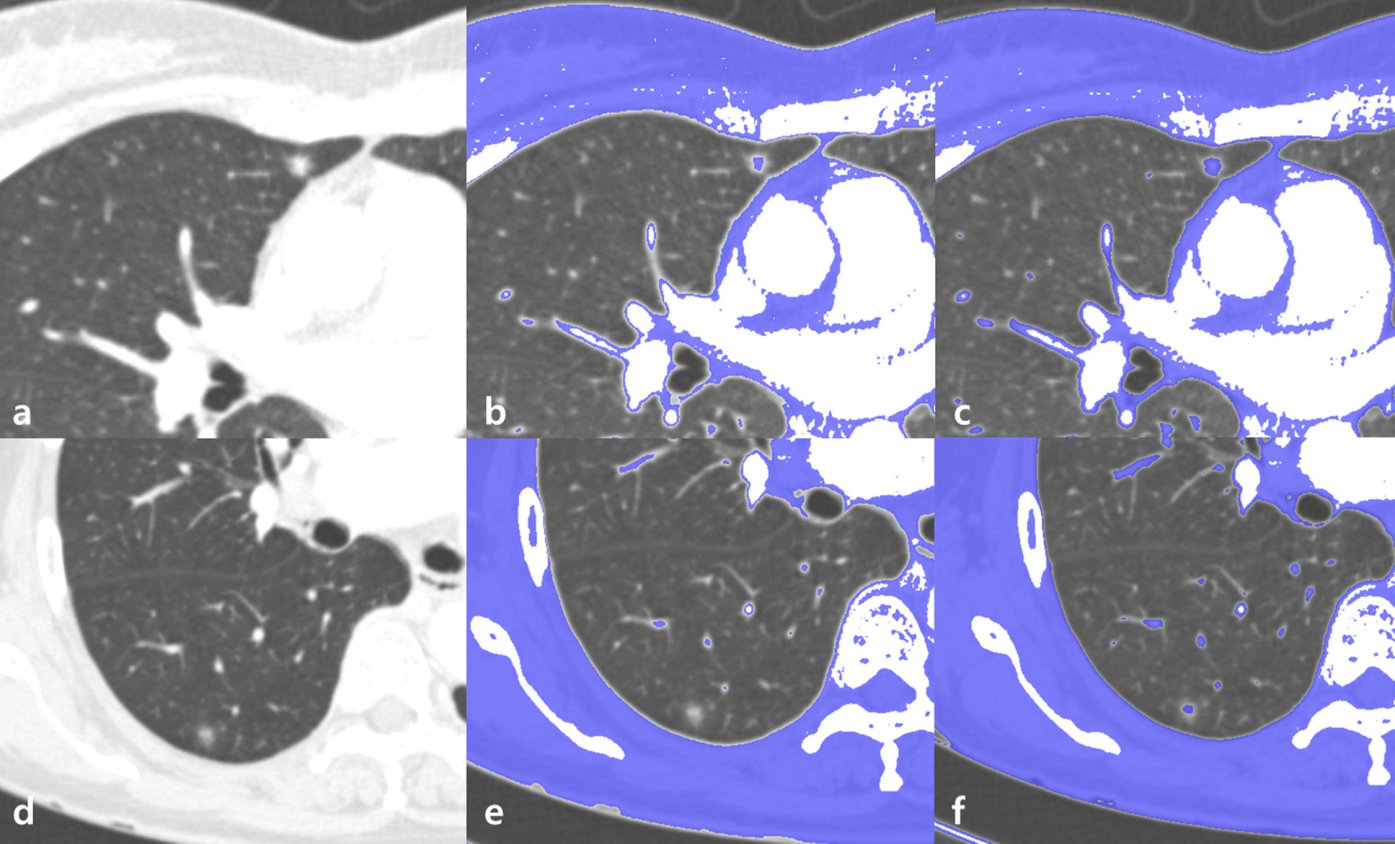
Different subcategorization of ground-glass opacity nodules (GGNs) according to different thresholds: Classifying GGN according to two thresholds. (a) Axial computed tomography (CT) image revealing GGN in the right middle lobe. (b) Axial CT images demonstrating areas above the high threshold (-160 Hounsfield units [HU]) (blue area). The GGN is identified as a part-solid GGN with a solid portion inside. (c) Axial CT images demonstrating areas above the low threshold (-400 HU) (blue area). The GGN is identified as a part-solid GGN with a solid portion inside. (d) Axial CT image demonstrating another GGN in the right lower lobe. (e) Axial CT images demonstrating areas above the high threshold (-160 HU) (blue area). The GGN is identified as a pure GGN without a solid portion inside. (f) Axial CT images demonstrating areas above the low threshold (-400 HU) (blue area). The GGN is identified as a part-solid GGN with a solid portion inside. As a result, the GGN in (a) is a part-solid GGN in both thresholds, whereas the GGN in (d) is a pure GGN in high threshold and part solid GGN in low threshold.
